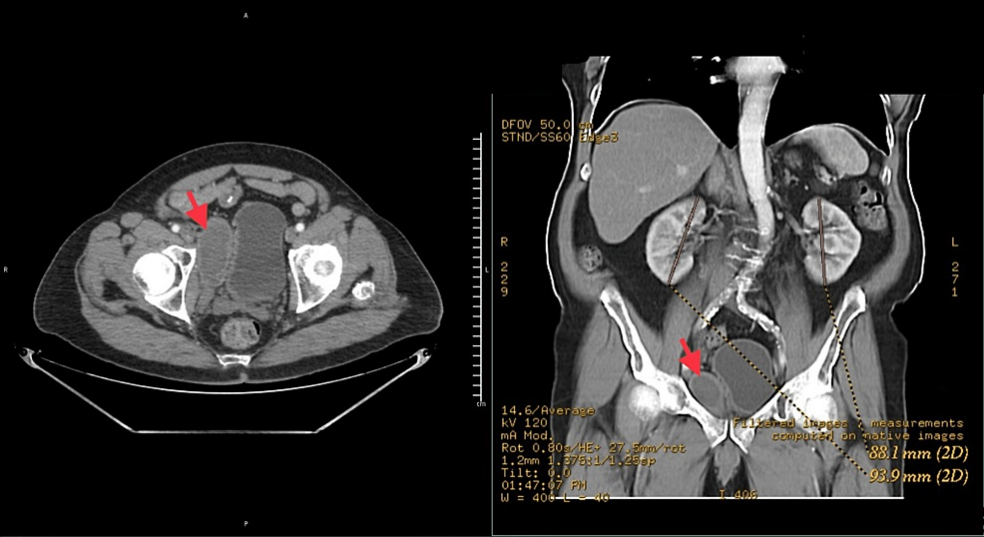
Pelvic CT scan 12 weeks after UroLift showing a 6.8 cm x 3.4 cm mass resolving right-sided pelvic hematoma.
